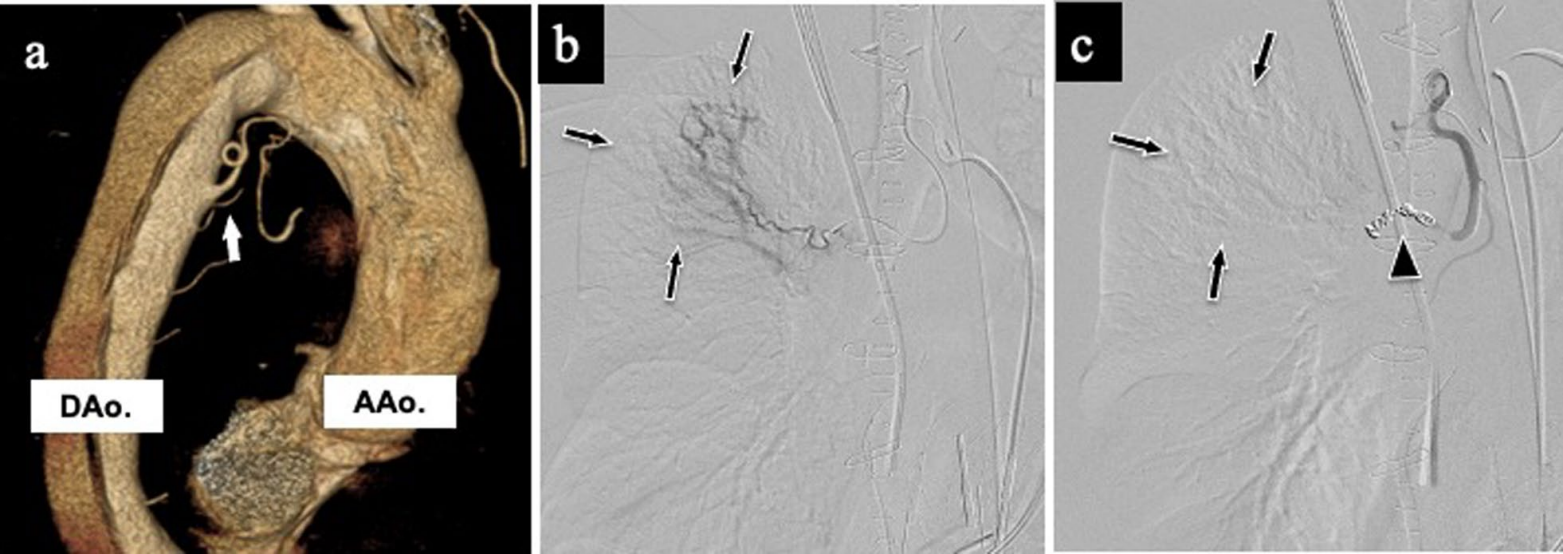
Preoperative computed tomography and postoperative angiography images in case 1: a BAH identified on preoperative three-dimensional CT image (white arrow); b pulmonary parenchymal blush in the right upper lobe demonstrated on BA angiography (arrows); c disappearance of parenchymal blush (arrows) after embolization (arrowhead) of the BA. *BAH* bronchial artery hypertrophy, *CT* computed tomography, *BA* bronchial artery, *AAo* ascending aorta, *DAo* descending aorta
